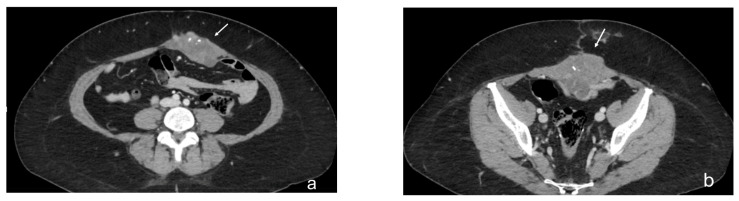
Contrast-enhanced CT scan, axial views (a,b). Case of a mass-like pattern of peritoneal carcinomatosis. Nodule (white arrow) located in the left rectus muscle of the abdomen, as a result of the confluence of multiple nodular lesions.
